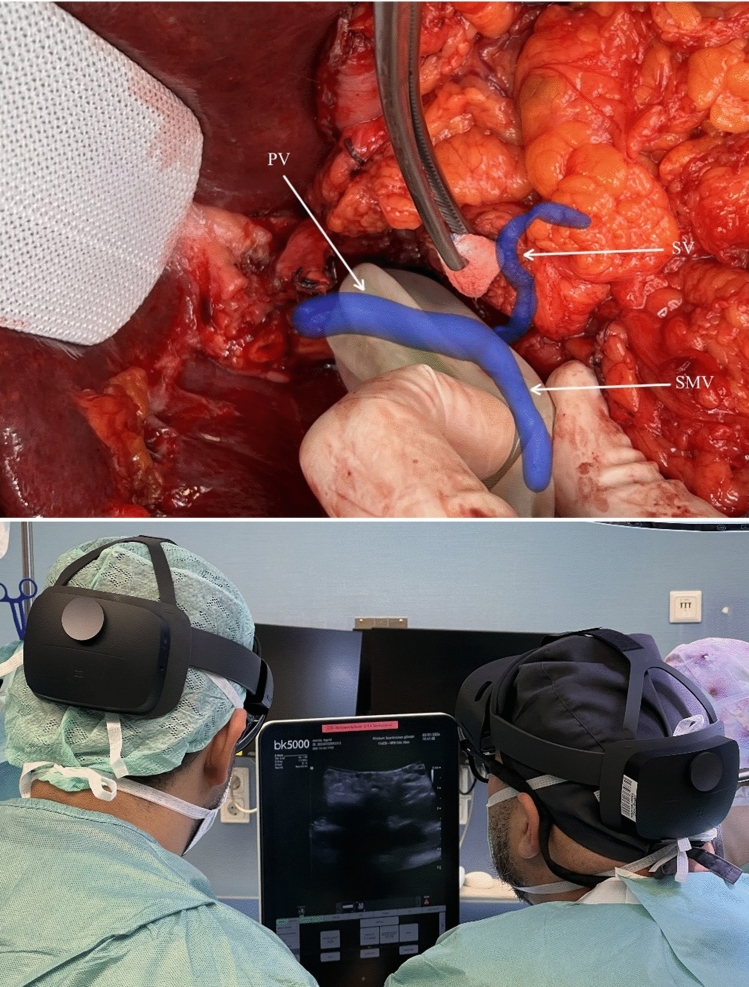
Intraoperative evaluation of the location and precision of the registered 3D anatomy using ultrasonography. Portal vein, PV; splenic vein, SV; superior mesenteric vein, SMV
